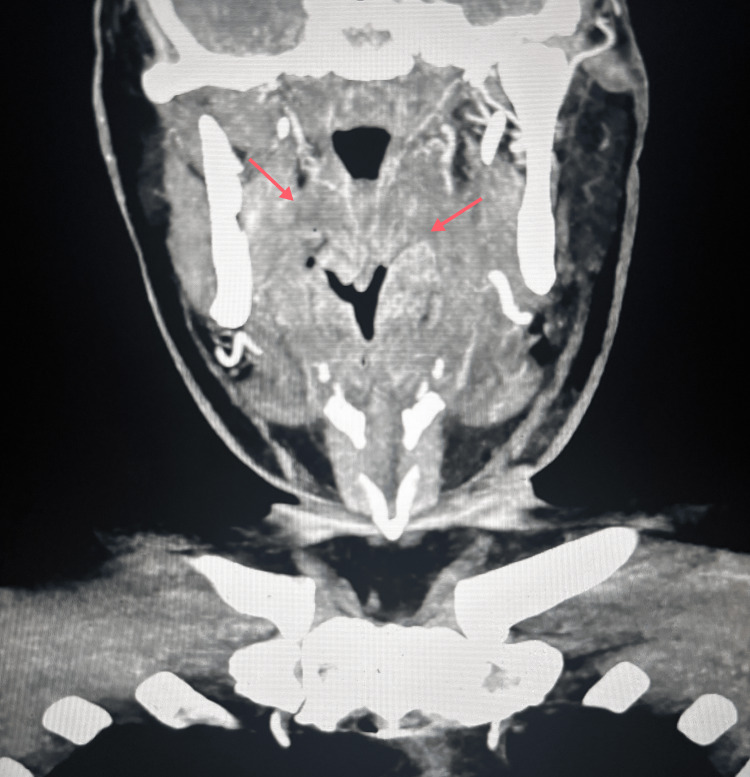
Coronal contrast-enhanced CT of the neck demonstrating oropharyngeal soft tissue thickening Coronal contrast-enhanced CT image of the neck demonstrating asymmetric soft tissue thickening centered in the left oropharynx (red arrows), involving the region of the uvula and epiglottis, with associated airway narrowing. CT: computed tomography
